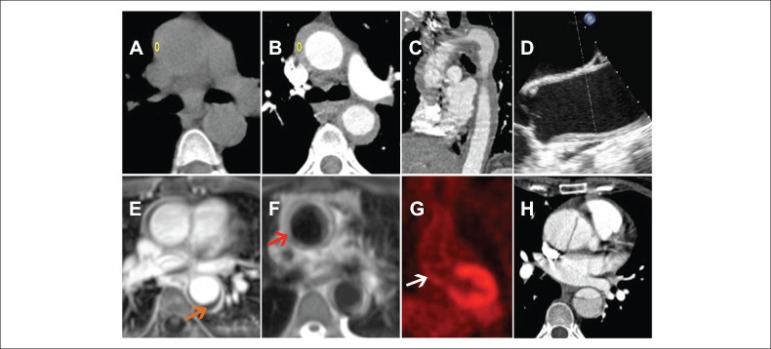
A) Non-contrast computed tomography showing low-attenuation concentric mural
